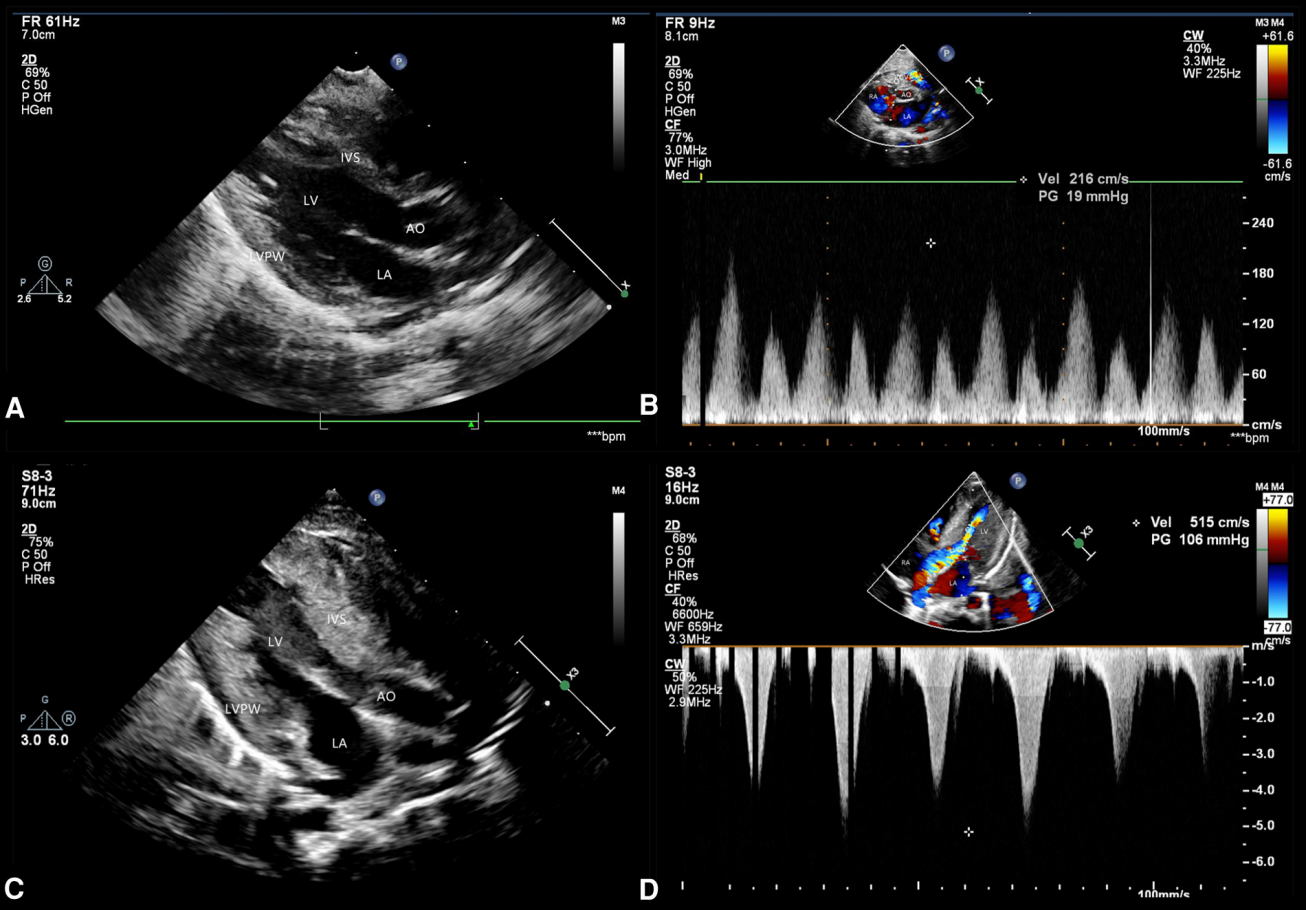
Echocardiographic findings. Echocardiography performed at the age of 3 months shows (A) a normal ventricular wall thickness but (B) slightly accelerated blood flow in the right ventricular outflow tract. Echocardiography performed at 5 months of age shows (C) significant myocardial hypertrophy and (D) an obstructive pattern in the left ventricular outflow tract.
